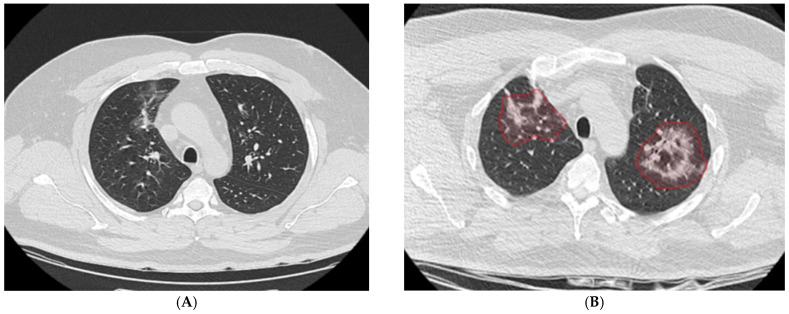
Progressive pulmonary changes on chest CT imaging in a 45-year-old patient with diffuse large *B-cell non-Hodgkin lymphoma*. (A) Baseline chest CT scan showing discrete bilateral ground-glass opacities and mild peribronchovascular thickening, with limited parenchymal involvement. (B) Follow-up chest CT scan demonstrating marked progression to bilateral consolidations with central ground-glass opacities and peripheral reticulation, highlighted in red, predominantly affecting the upper lobes.
